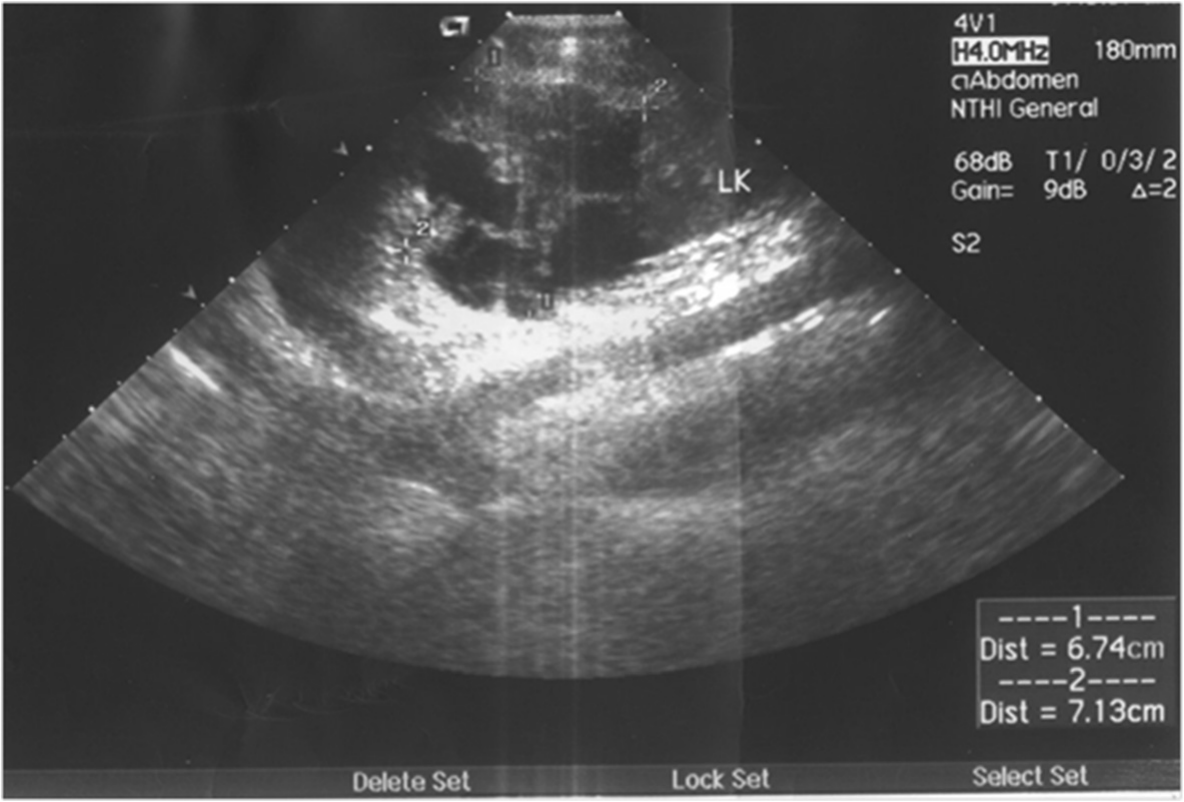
A typical B ultasonographic image of CRCC. The uneven cystic walls, hyperechoicsepa, and nodules were visible.
